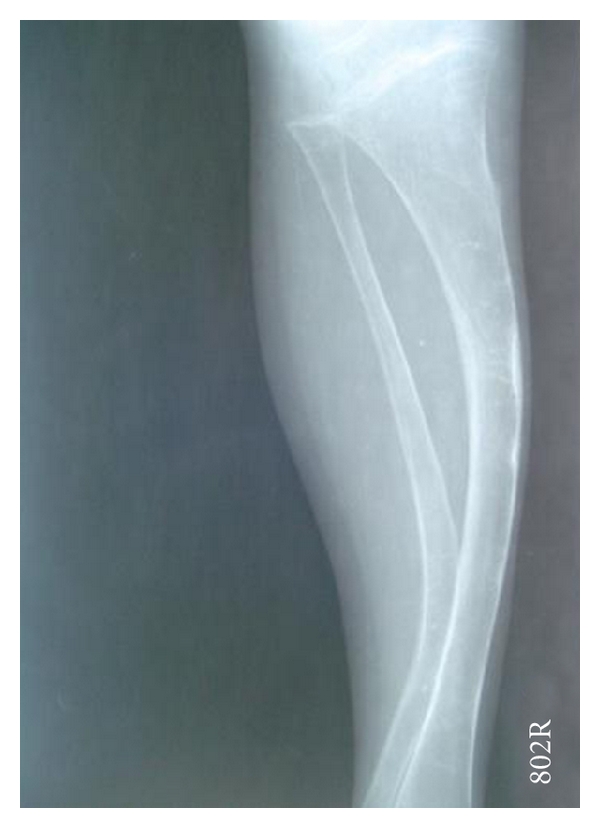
Radiograph showing bowing tibia and fibula.
